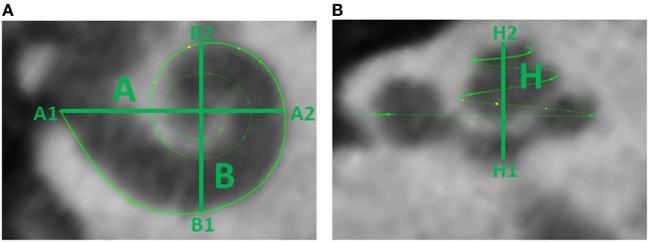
(A) Cochlear dimensions estimated from CBCT. *A* and *B* values in CBCT data as clinically derived measures. (B) Height (*H*) of the cochlea starting from the lowest basal point to the apex (Helicotrema).
